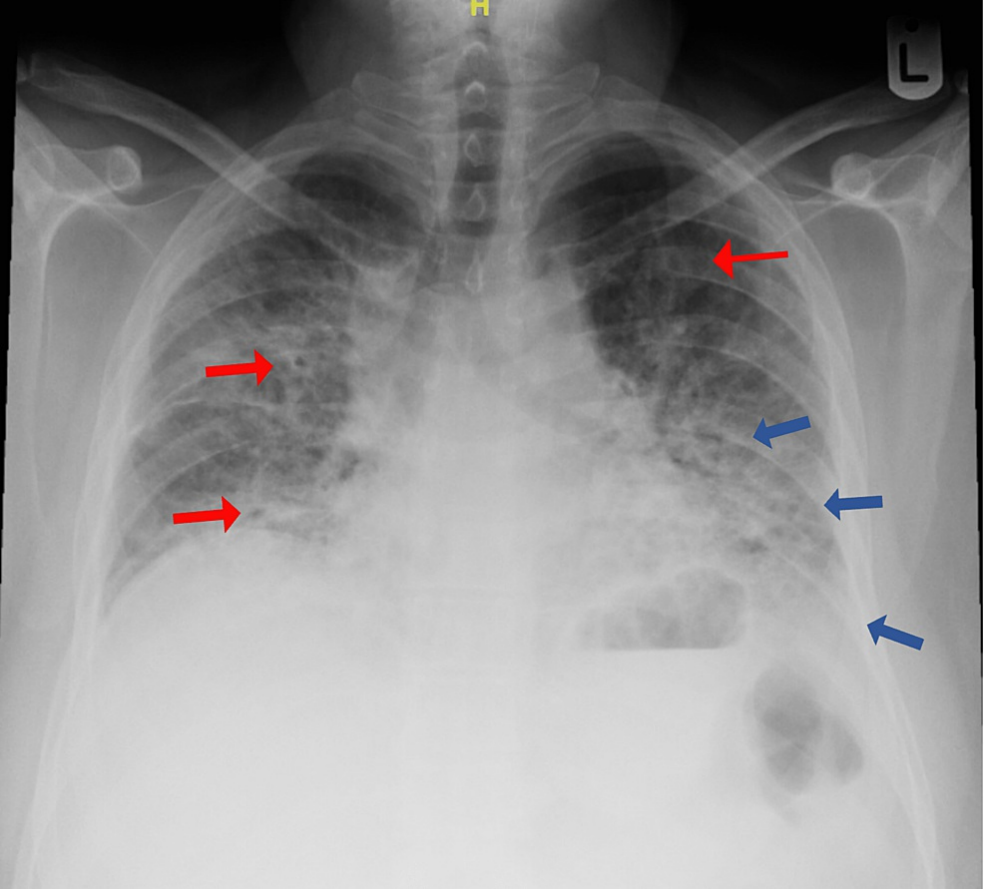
Patient’s chest x-ray showing bilateral cystic changes (red arrows) with patchy airspace opacities (blue arrows) mostly appreciated in the left lower lobe and left retrocardiac area
